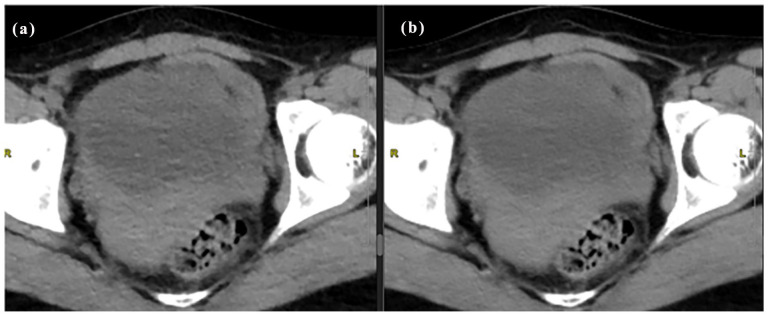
Idose4 (a) versus Precise Image (b) for unenhanced abdomen and pelvis CT.
